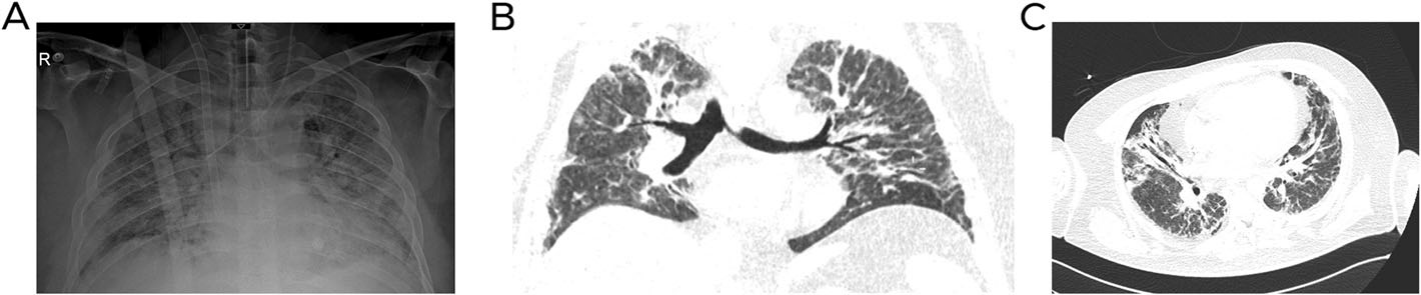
Forty-five-year-old man without comorbidities with H1N1 virus infection who was admitted to the intensive care unit with acute respiratory distress syndrome. a Anteroposterior chest radiography demonstrates bilateral diffuse pulmonary infiltrates and consolidations. b and c Chest computed tomography on coronal and axial planes also show consolidations and bilateral ground-glass opacities
